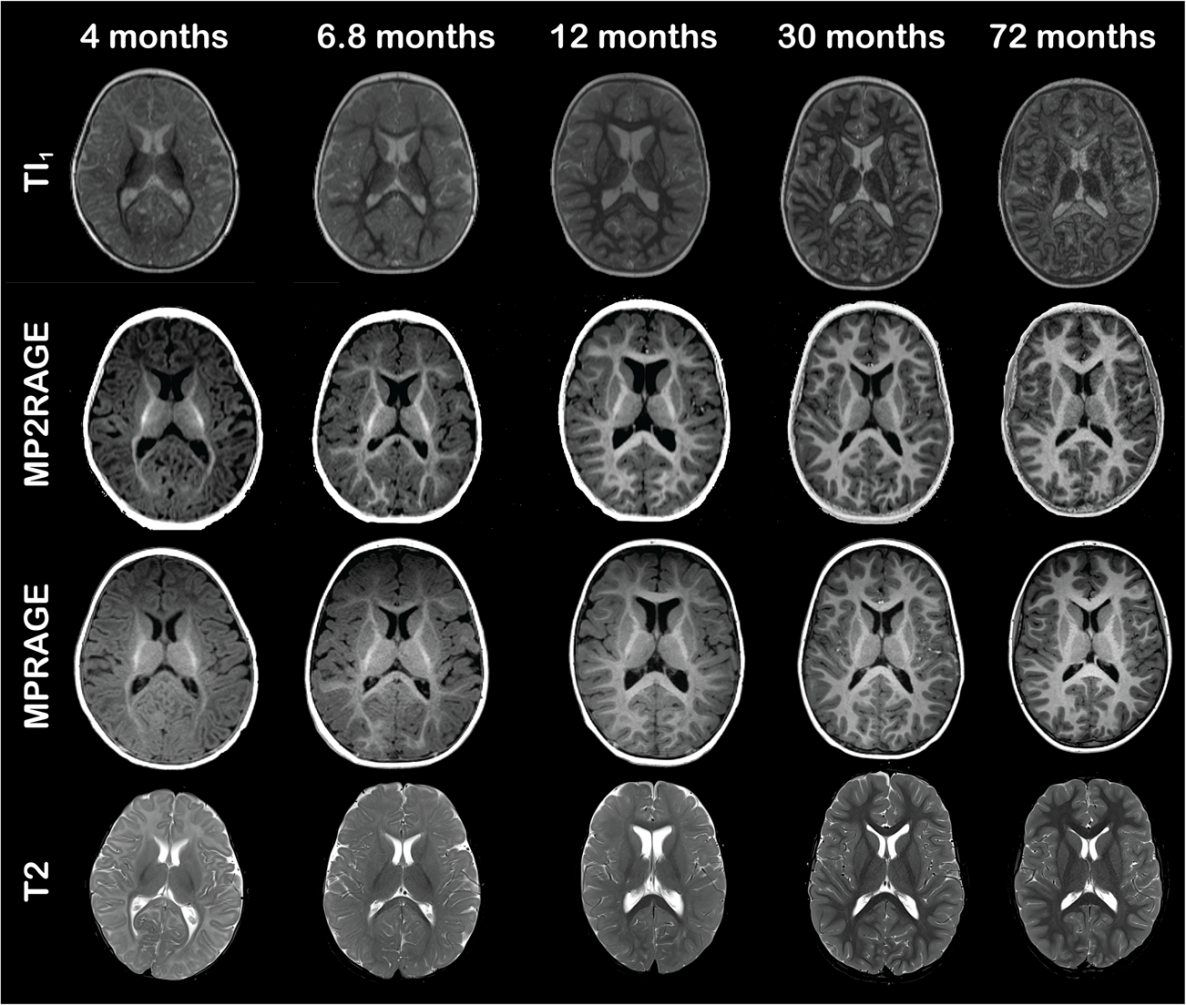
Axial TI_1_, MP2RAGE (magnetization-prepared 2 rapid acquisition gradient echoes), MP-RAGE (magnetization-prepared rapid acquisition gradient echo) and T2-weighted images at the level of the posterior limb of the internal capsule for five MRI-negative children (boys ages 4 months, 6.8 months and 30 months, and girls ages 12 months and 72 months)
